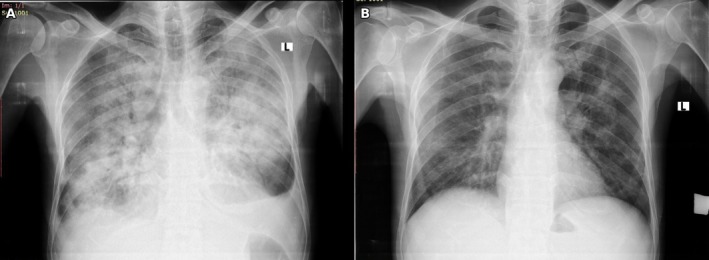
Chest radiography at admission (A) showing bilateral diffuse perihilar fluffy air‐space opacities with air bronchograms and blunting of left costophrenic angle, consistent with acute pulmonary edema, and at discharge (B) demonstrating near‐complete resolution of these findings following treatment.
